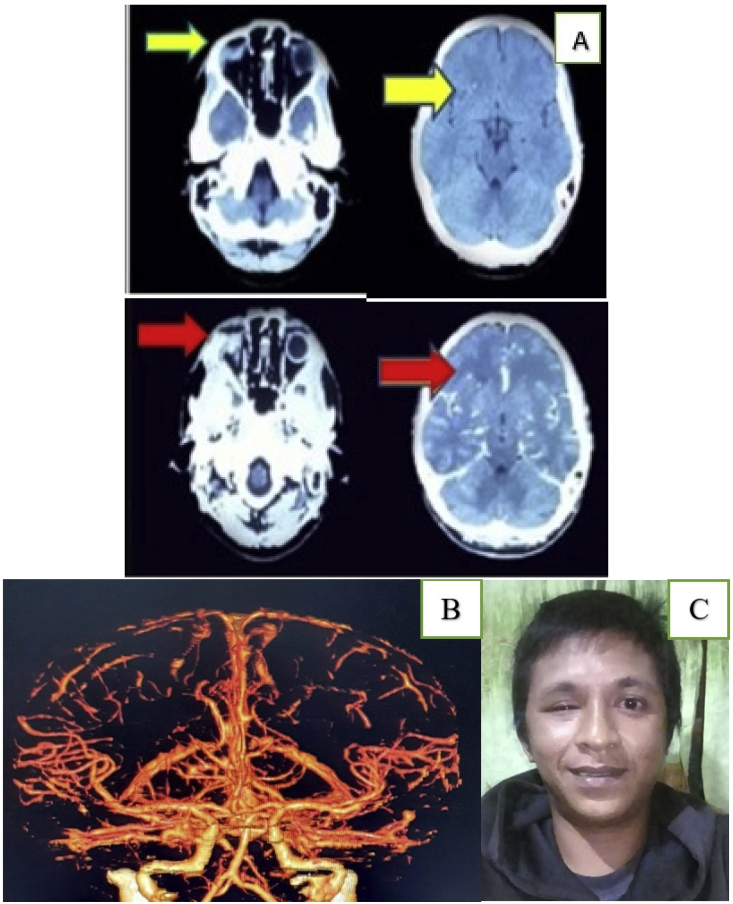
The post-operative evaluation six months later A). A CT brain scan with (red arrow) and without contrast (yellow arrow) presented a slight ischemia in the foreign-body track (right frontal lobe) and a globe rupture on the right eye. B). A CTA does not appear to show abnormal vascular brain structures. C). Enophthalmos in the patient's right eye. (For interpretation of the references to colour in this figure legend, the reader is referred to the Web version of this article.)
